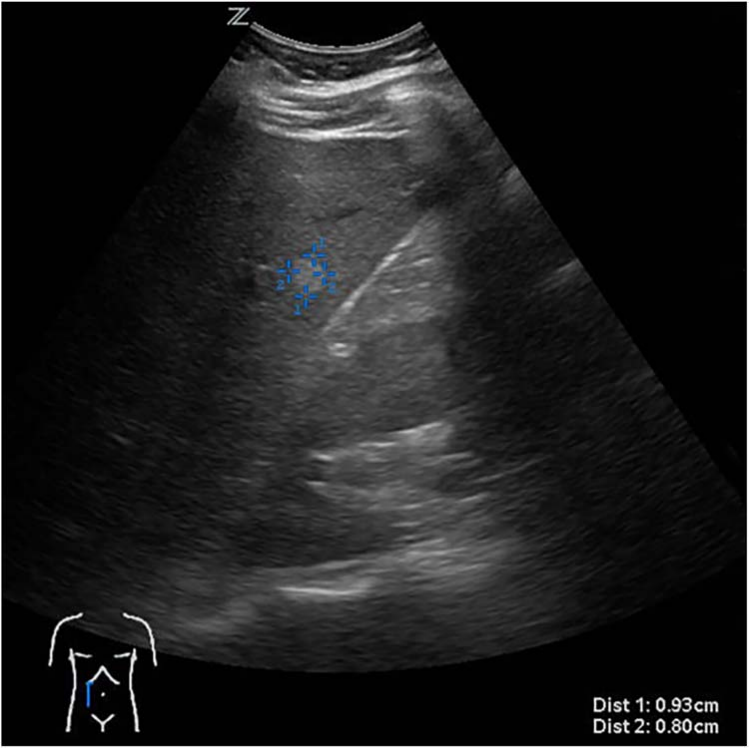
First documentation of liver cyst of the patient in ultrasound.
